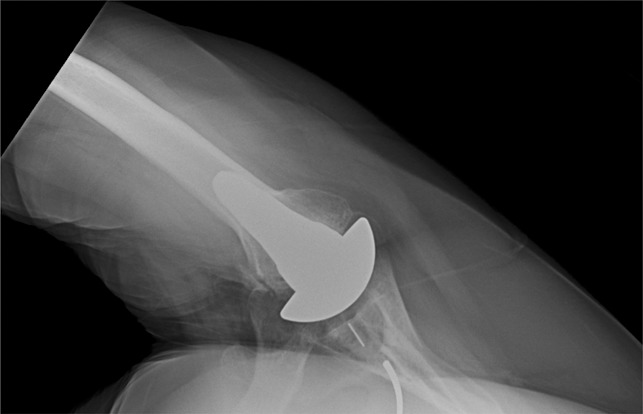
Radiograph showing the example of a nondisplaced, nonunion of a lesser tuberosity osteotomy.
